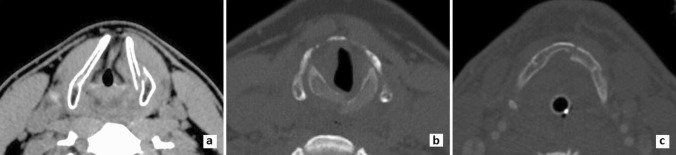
CT scans of patients with laryngeal traumas. A A displaced thyroid fracture in a 26-year-old male following suffocation on assault. B A displaced cricoid fracture in a 62-year-old female from an unintentional fall. C Hyoid fracture in a 56-year-old male in result of a motor vehicle incident
